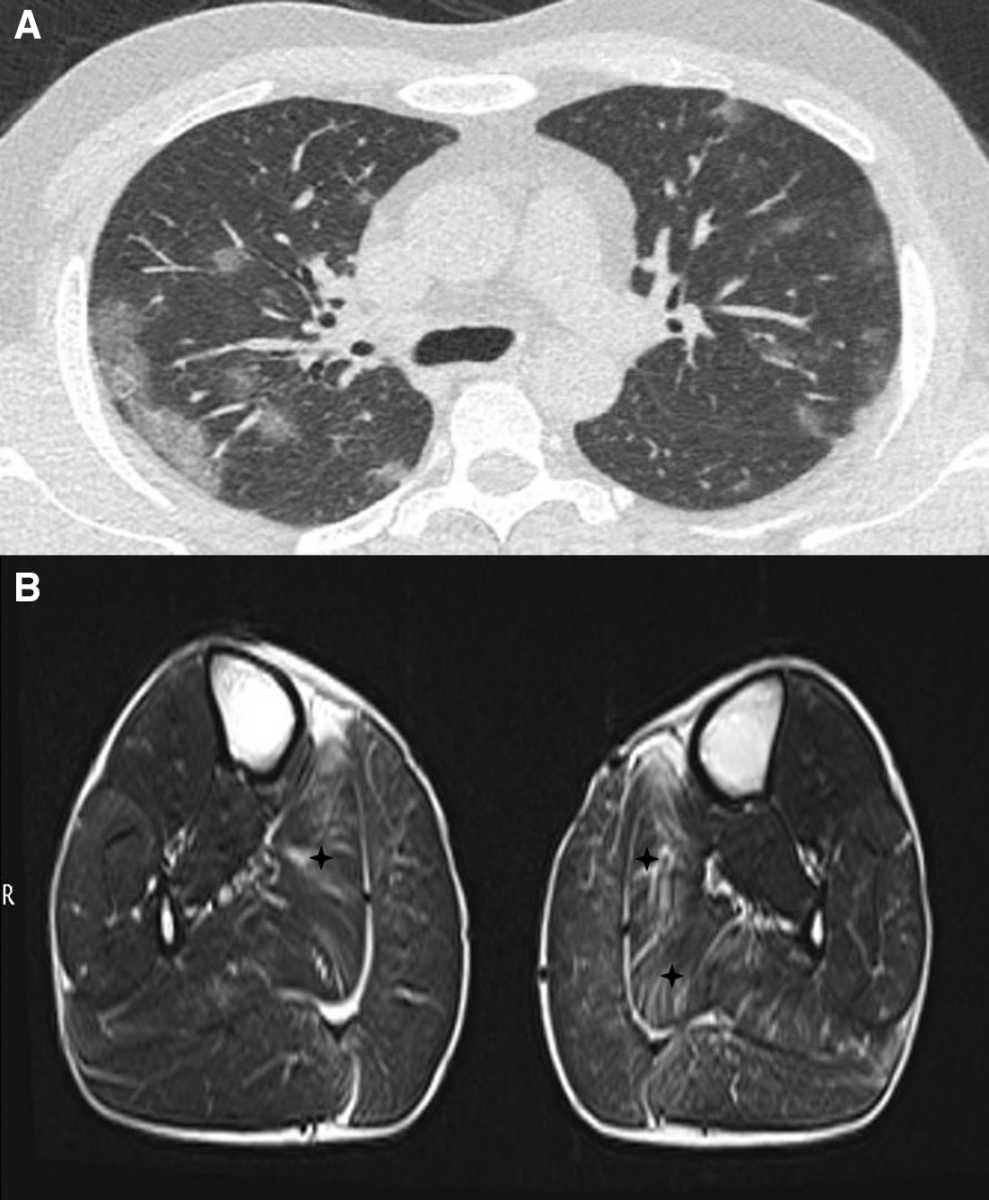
(A) CT scan showing ground-glass opacities and (B) MRI in T2 short-tau inversion recovery sequence showing bilateral gastrocnemius muscle oedema (asterisk).
